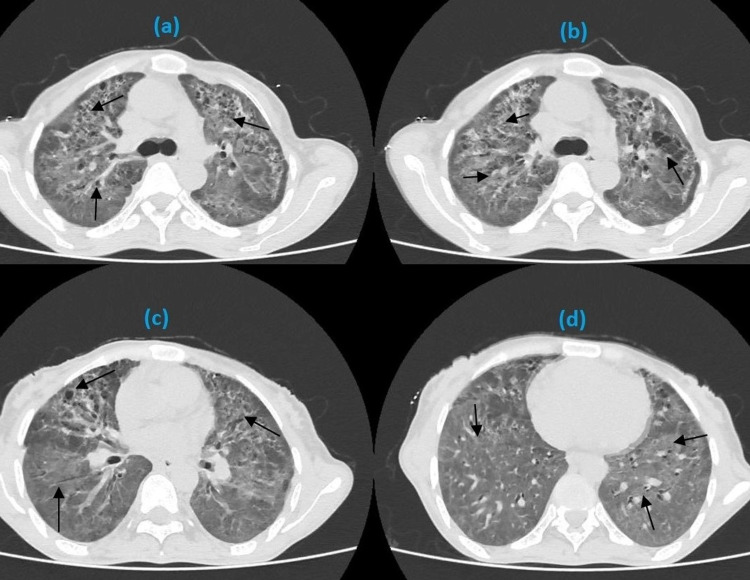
HRCT showing diffuse ground-glass haze along with bronchiectasic changes predominantly in the mid zones (a, b), and multiple cystic lesions in all zones of both lungs (a-d). HRCT; high-resolution computed tomography.
